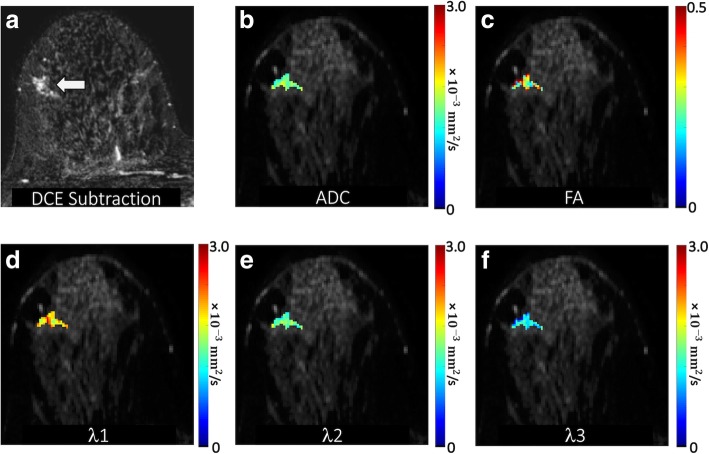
Benign non-mass enhancement detected in a 40-year-old patient undergoing MRI to evaluate newly diagnosed cancer. a DCE post-contrast image demonstrates a 20-mm focal heterogeneous non-mass enhancement in the middle right breast 9 o’clock (arrow), assigned a BI-RADS category 4. DTI-derived parametric maps of b apparent diffusion coefficient (ADC), c fractional anisotropy, and eigenvalues d
